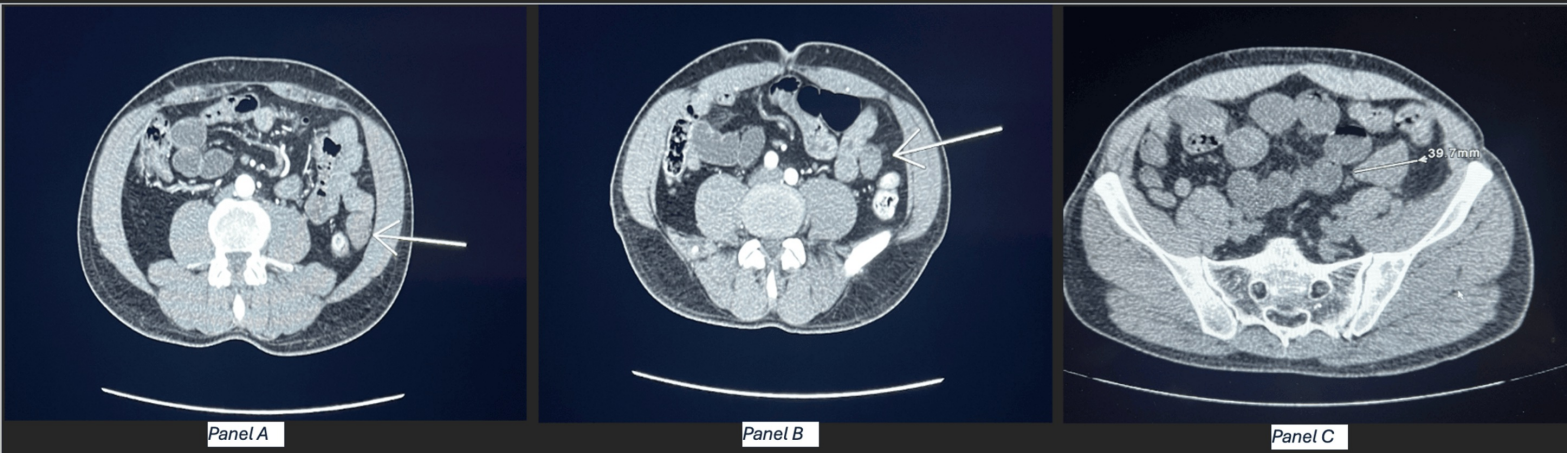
CT scan with panels A, B, and C (left to right) Panel A: Axial CT of the abdomen. The arrow points to mass A in the left lower quadrant. Panel B: Axial CT of the abdomen. The arrow points to mass B in the left lower quadrant. Panel C: Axial CT of the abdomen. Measurement of mass C in the left lower quadrant is shown.
